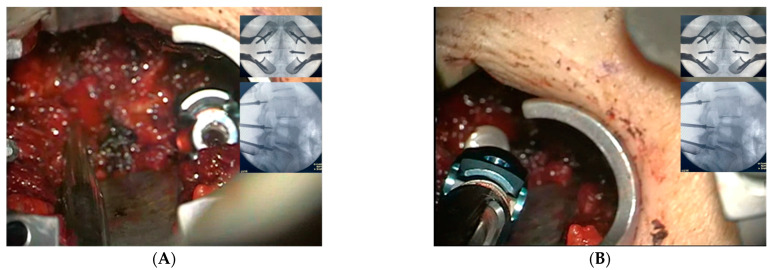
AP and lateral radiographs are saved in a fluoroscopic screen to aid the insertion of the pedicle screw utilizing the standard freehand technique. (A) Pedicle finder insertion using a trajectory based on the saved radiographs. (B) Pedicle screw insertion.
